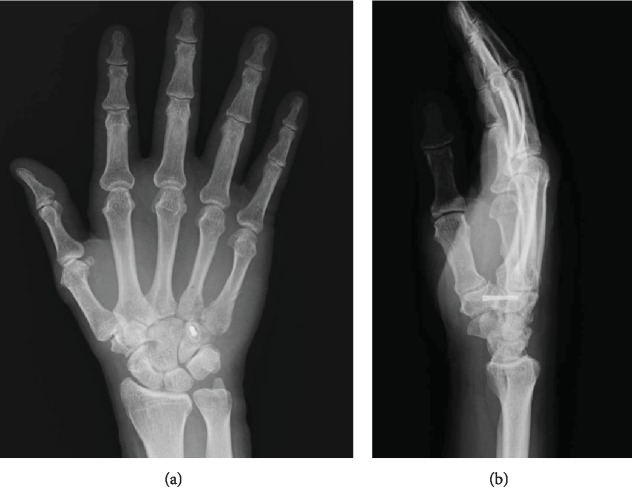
Radiographs after the removal of the K-wires showing the maintenance of the reduction of CMC joint. (a) Posteroanterior view. (b) Oblique semisupinated view.
